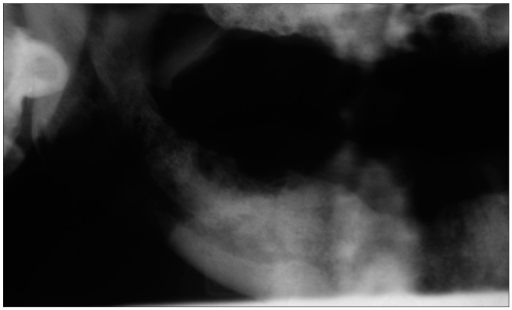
(b) Panoramic radiograph showing osteradionecrosis
